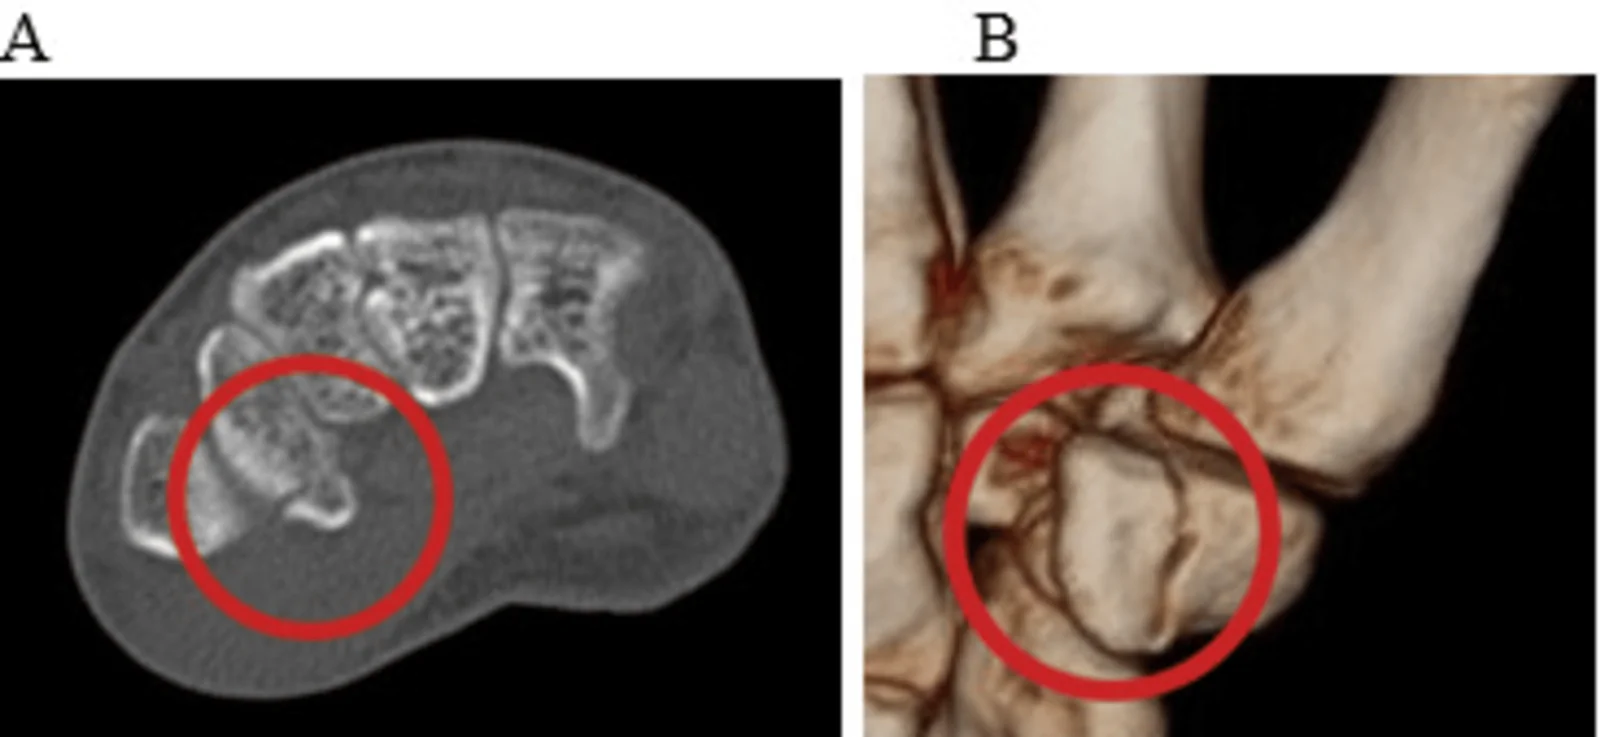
Case 1: Initial computed tomography (CT) findings. Axial (A) and three-dimensional (B) CT images showing a non-displaced fracture of the trapezial tubercle (red circles)．
